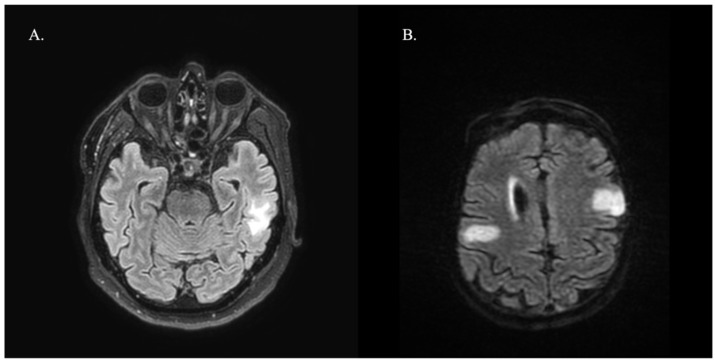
T2-weighted brain MRI with fluid-attenuated inversion recovery (FLAIR) hyperintense lesions attributable to autoimmune encephalitis related to anti-GAD65. (A) A patient with a temporal lesion involving the white matter that clinically presented with limbic encephalitis. (B) Parietal and frontal tumefactive lesions in a patient presenting with epilepsy.
